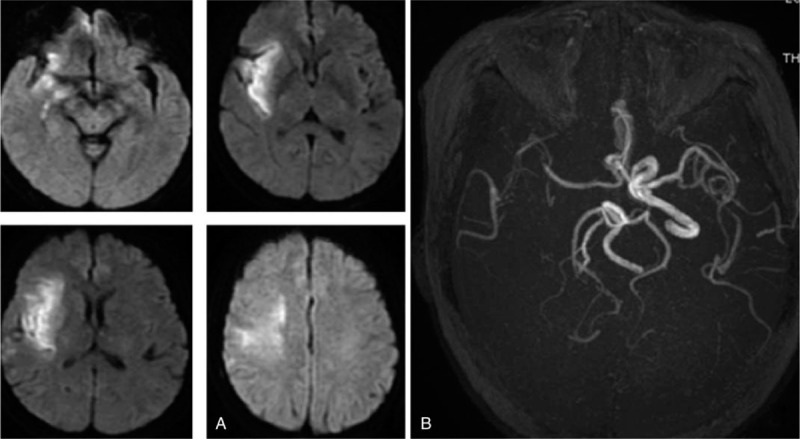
Imaging studies. (A&B) Cranial magnetic resonance imaging in patient showed acute multiple cerebral infarction, most of the right internal carotid artery not shown exactly; the right middle cerebral artery imaging shallow.
